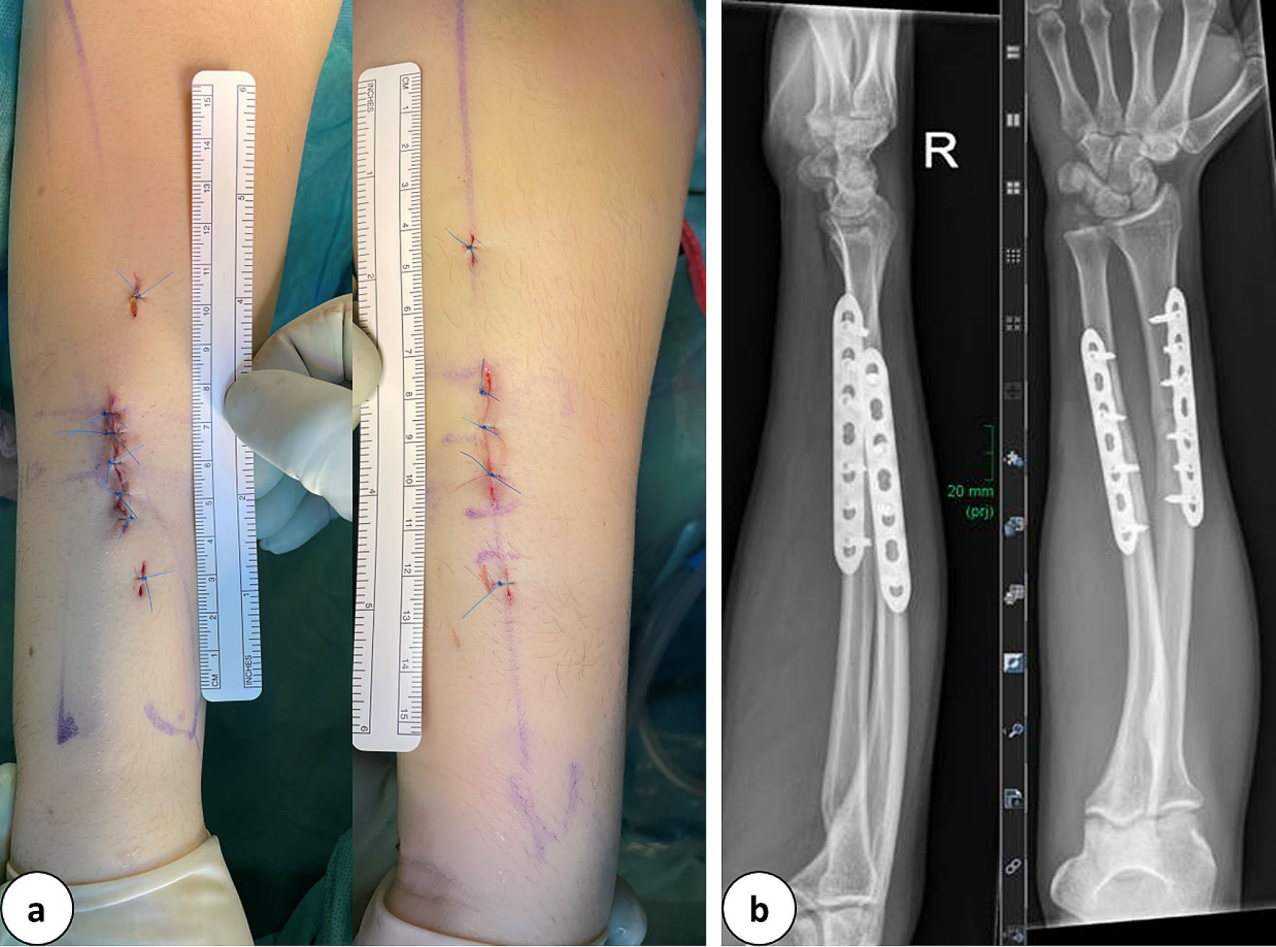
a: Clinical depiction of minimally invasive incisions (3.5 cm) and stab incisions executed in the scenario of a forearm fracture (AO: 2R2A3, 2U2A3). b: Postoperative radiographic imaging captured in two dimensions, showcasing plate osteosynthesis and fracture realignment
